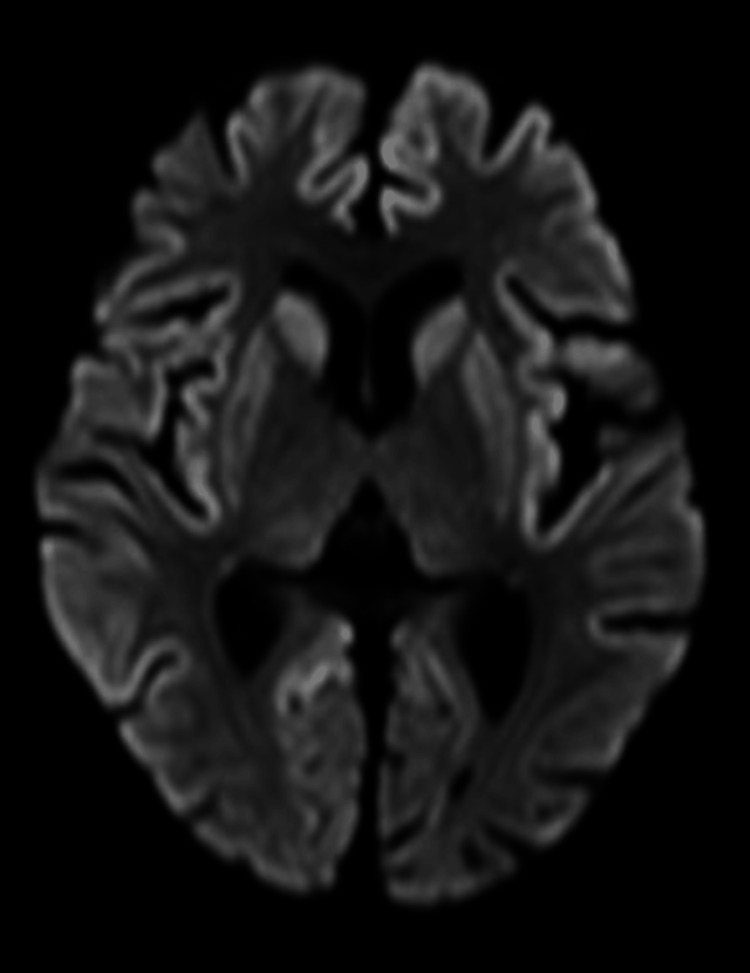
Brain MRI findings. Brain MRI revealed mild atrophy of the cerebrum and high signal levels in the cerebral cortex and basal ganglia on DWI.
